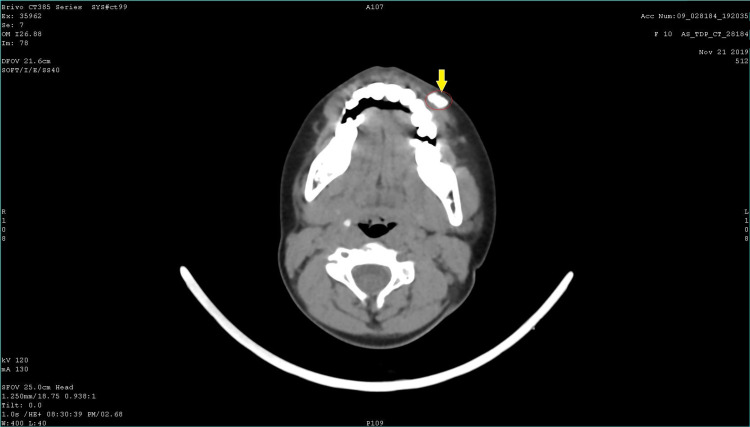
CT axial section showing a calcified mass at the region of the left upper lip (lesion encircled in red and denoted by the yellow arrow).
